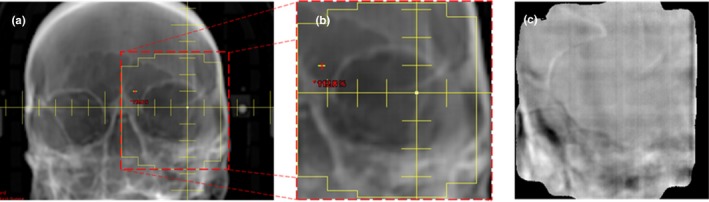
Digitally reconstructed radiograph (a), zoom‐in on the treated field (b), and relative difference (c) images are shown for one head and neck patient. The bones visible in the exit images could potentially be used to track intrafraction motion.
